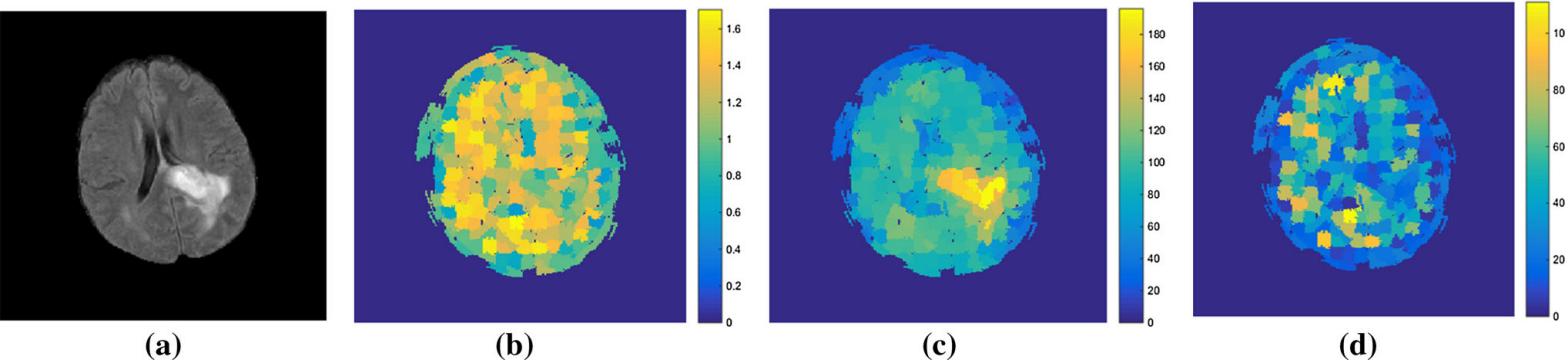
An example of fractal analysis applied to a grade III glioma to generate superpixel-based fractal feature maps: a FLAIR image, b area, c mean intensity and d fractal dimension
